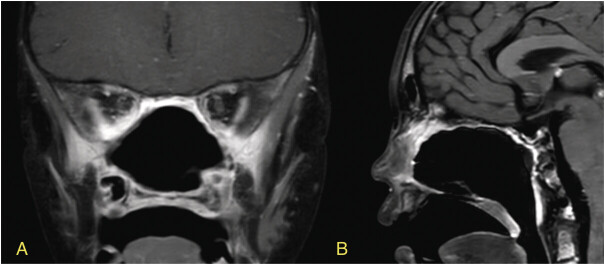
Postoperative MRI demonstrating the surgical cavity following definitive resection of a sinonasal tumor. Coronal (
